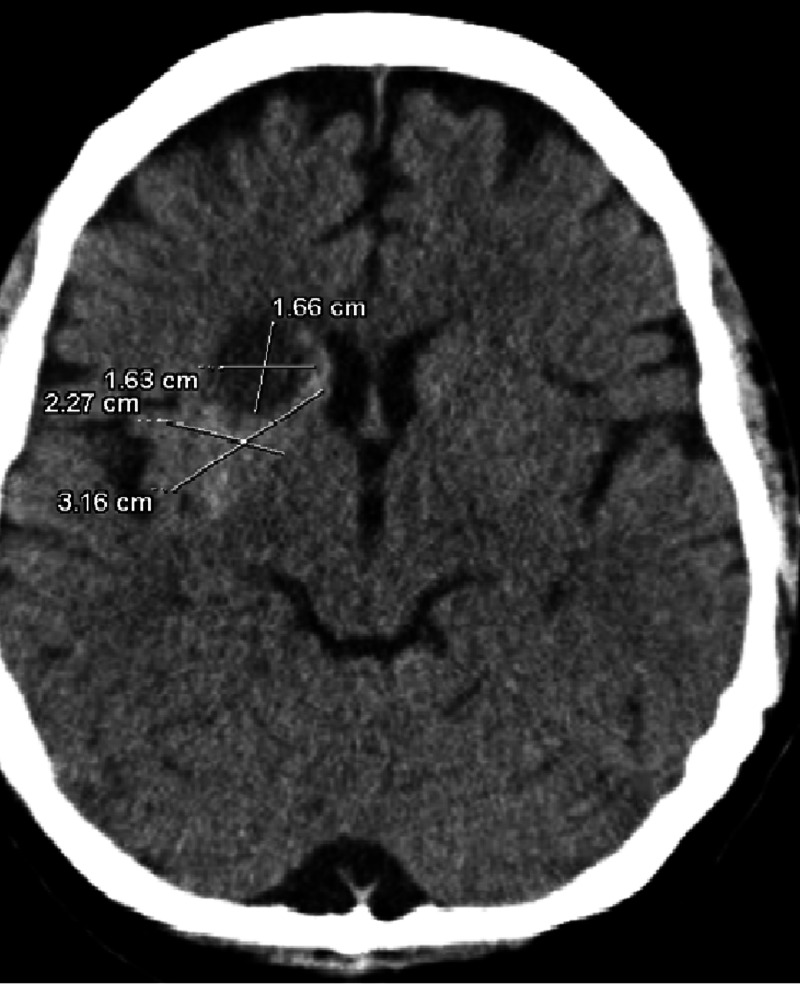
CT head shows hyperdense right caudate nucleus head and lentiform nucleus suggesting osmotic myelinolysis secondary to chronic uncontrolled non-ketotic hyperglycemia CT: computed tomography
